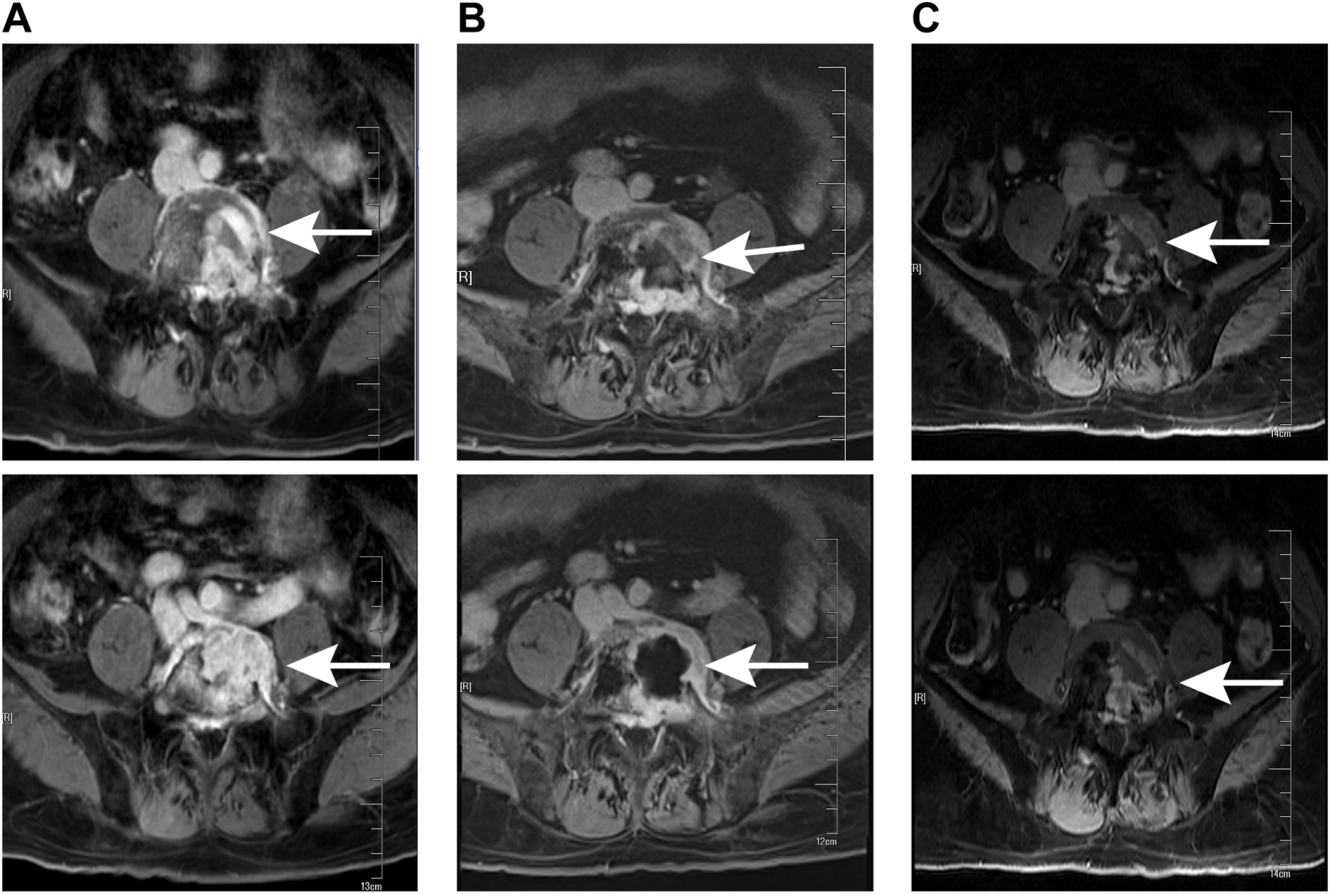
MRI examination at different stages of treatment. (A) Preoperative lumbar MRI showed a lesion in the L5 (white arrows). (B) MRI conducted prior to radiotherapy following the surgical operation revealed the lesion in the L5 (white arrows). (C) The latest follow-up MRI examination indicated that there was no recurrence of the L5 pyramidal lesion (white arrows).
